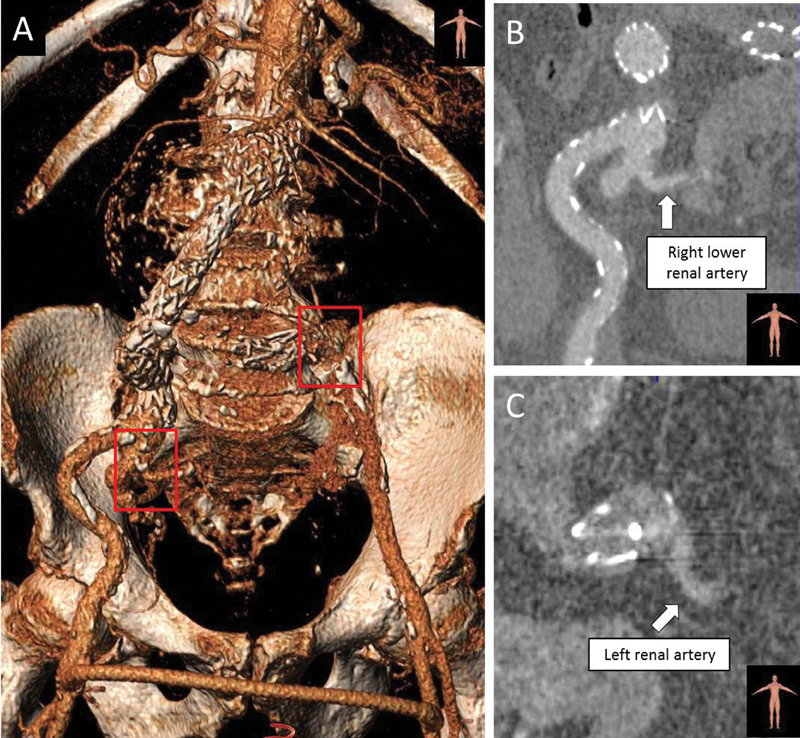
One-month computed tomography angiogram. (
